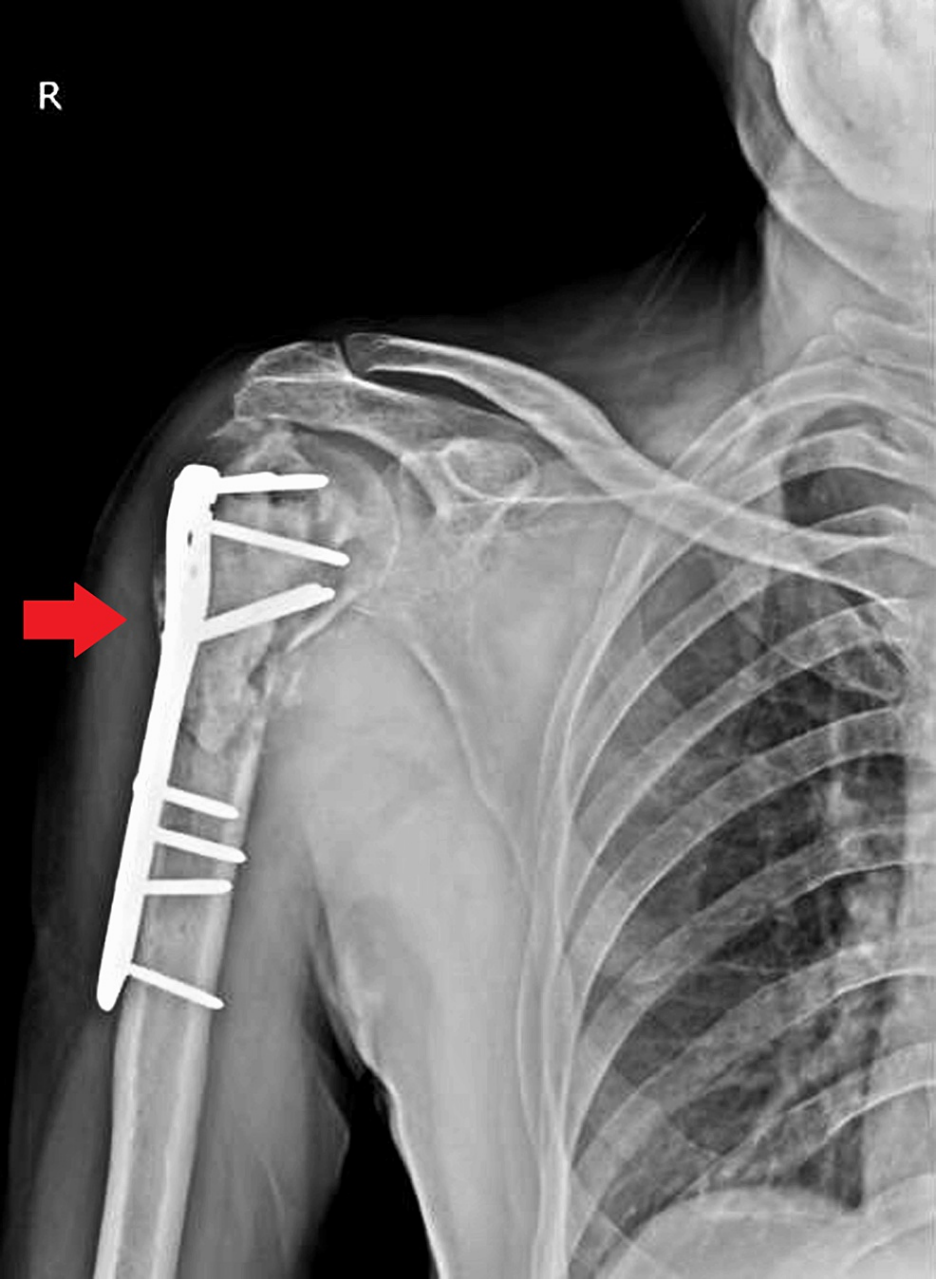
Post-operative X-ray of the right shoulder having a bone graft, a bone cement, and a PHILOS plate PHILOS: proximal humeral internal locking system
